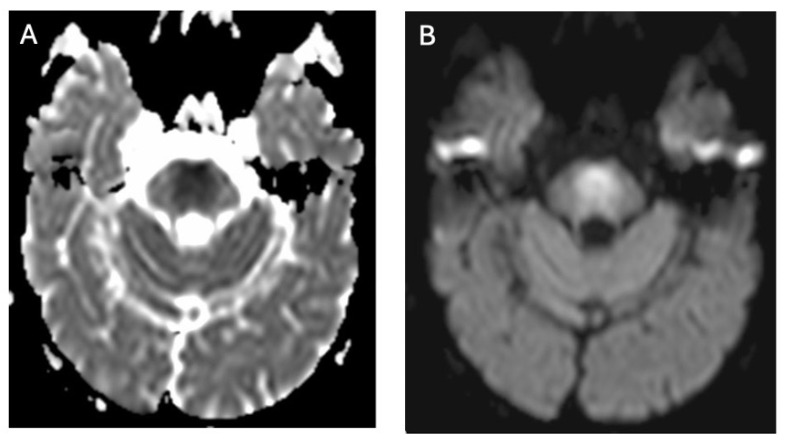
Neuroimaging findings of WD affecting the pons—a hypointense signal on ADC maps (A) and a hyperintense signal on diffusion-weighted imaging (DWI) (B), indicative of cytotoxic edema (own materials of the neurology department).
